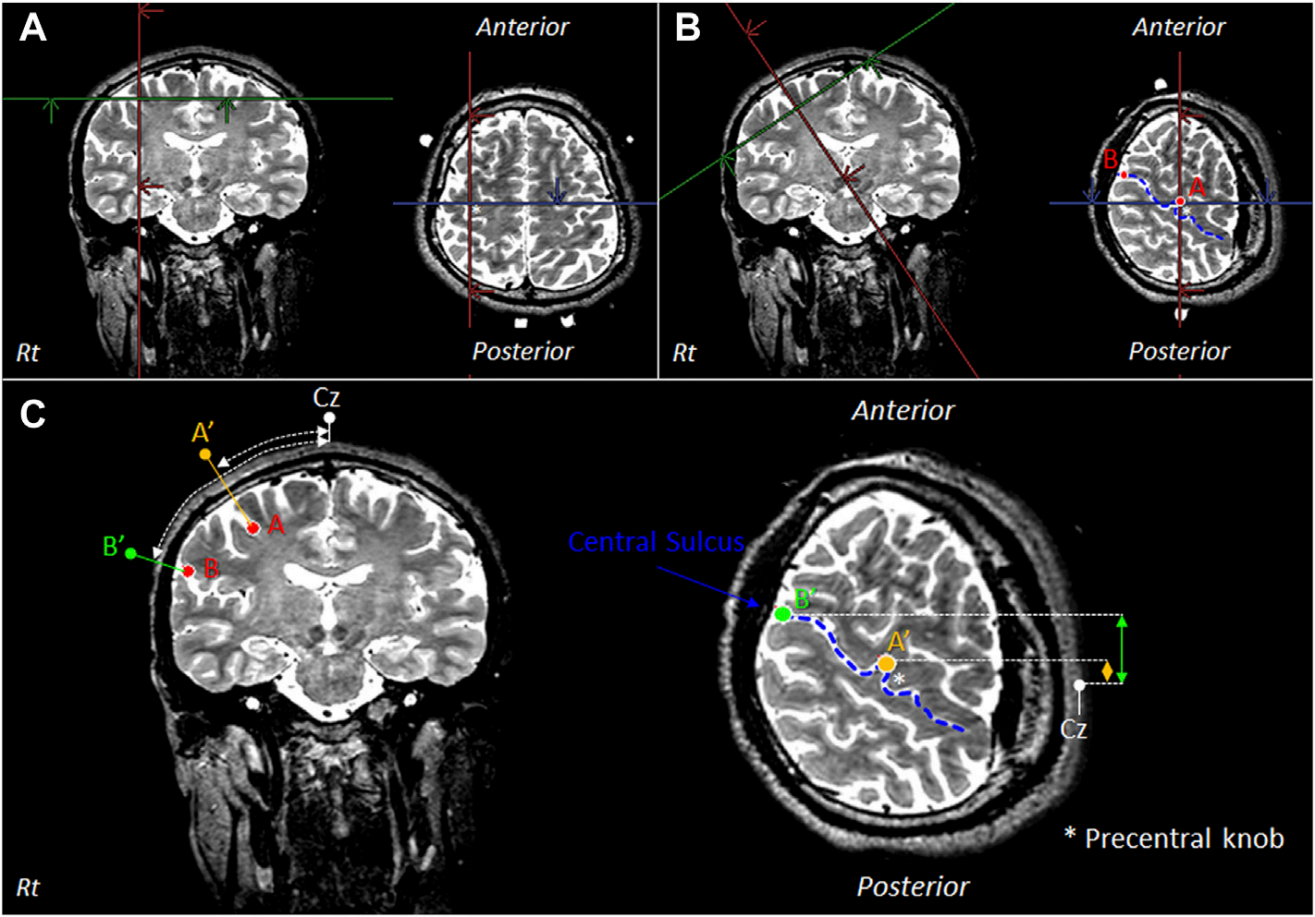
MRI for selecting the OMC measurement region. (A) Extract the outer edge of the precentral knob in the primary motor cortex (M1) and define it as point A. (B) Display an image in which point A is tilted as an axis perpendicular to the skull. In the displayed image, the outer edge of the central sulcus is defined as point B. The blue line indicates the central sulcus of the right hemisphere. (C) Points A′ and B′ are defined as points passing through points A and B and perpendicular to the skull. There is OMC in the area between points A′ and B′. Thus, the distances between Cz, point A′, and point B′ in the longitudinal direction and lateral direction were measured. OMC: oral motor cortex. Cz: location of vertex on the scalp. Rt: right.
